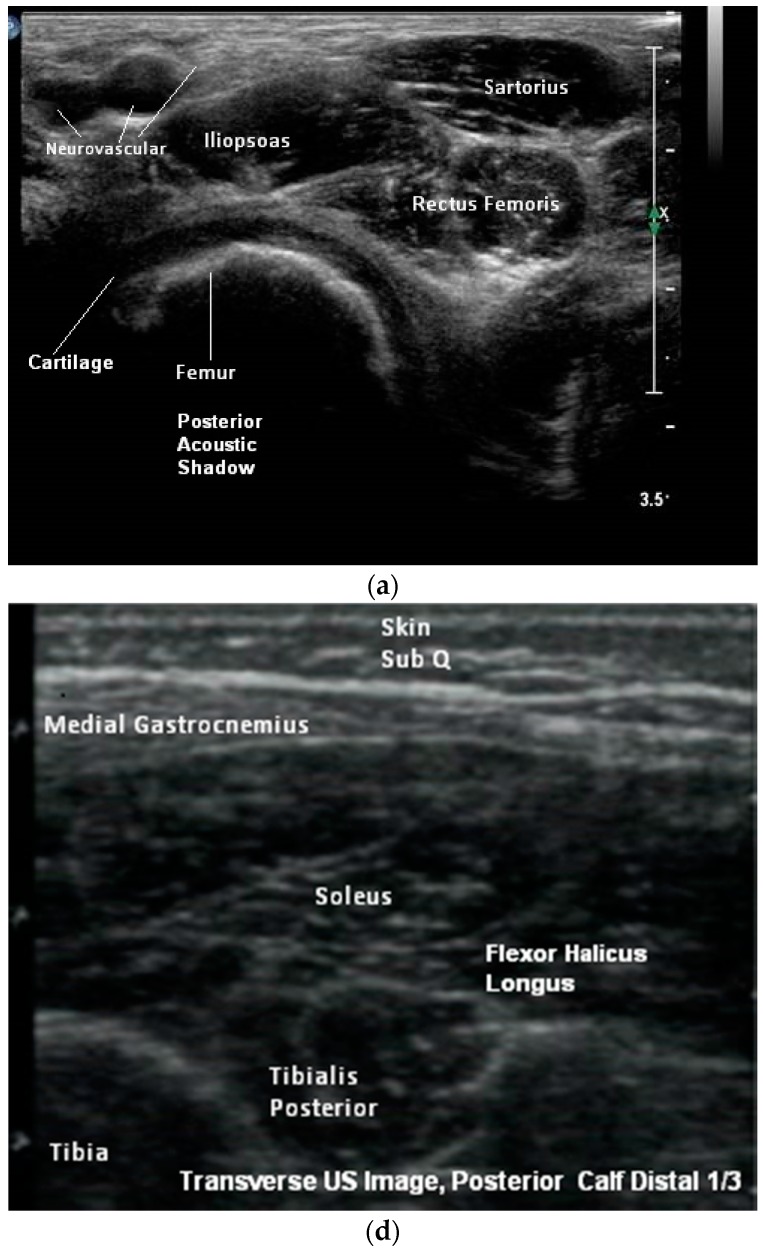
(a) Transverse B-mode ultrasound (US) Image, Proximal Thigh; (d) Transverse B-mode US Image, Posterior Calf (Distal 1/3).
